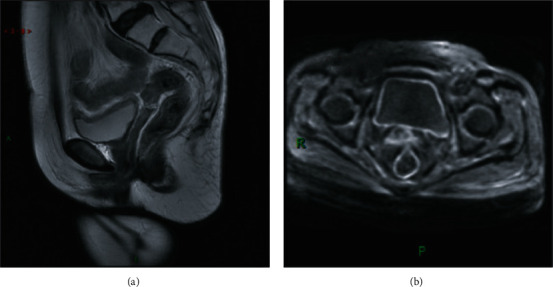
Patients with cervical squamous adenocarcinoma with MRI findings completely compatible with clinical examination.
